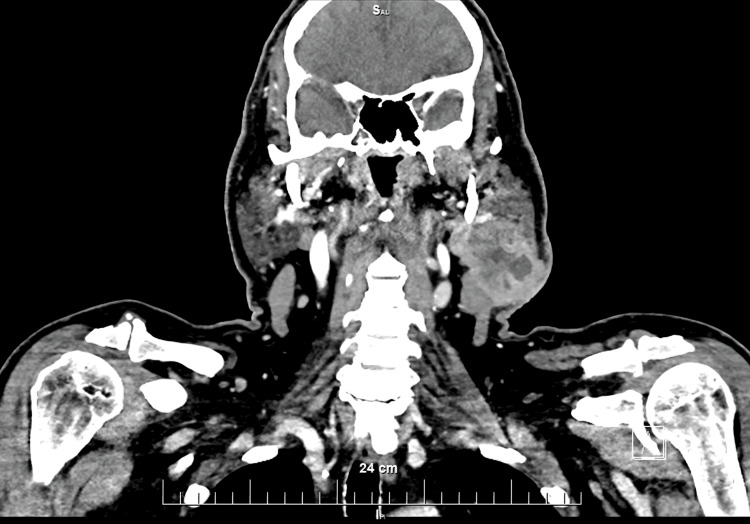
Axial CT scan of the head and neck showing the tumor mass in the left parotid gland. The tumor mass involves the two lobes of the left parotid gland and the facial nerve between them. The Ill-defined margins demonstrated by the lesion are strongly suggestive for malignancy.
